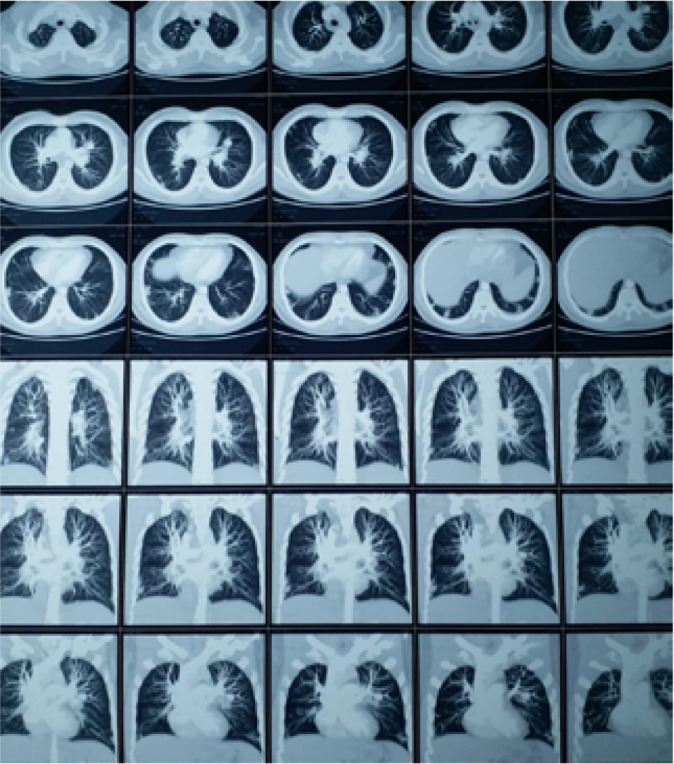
Radiographic presentation of Hughes-Stovin Syndrome. CT-Pulmonary Angiogram revealing pulmonary artery aneurysms.
